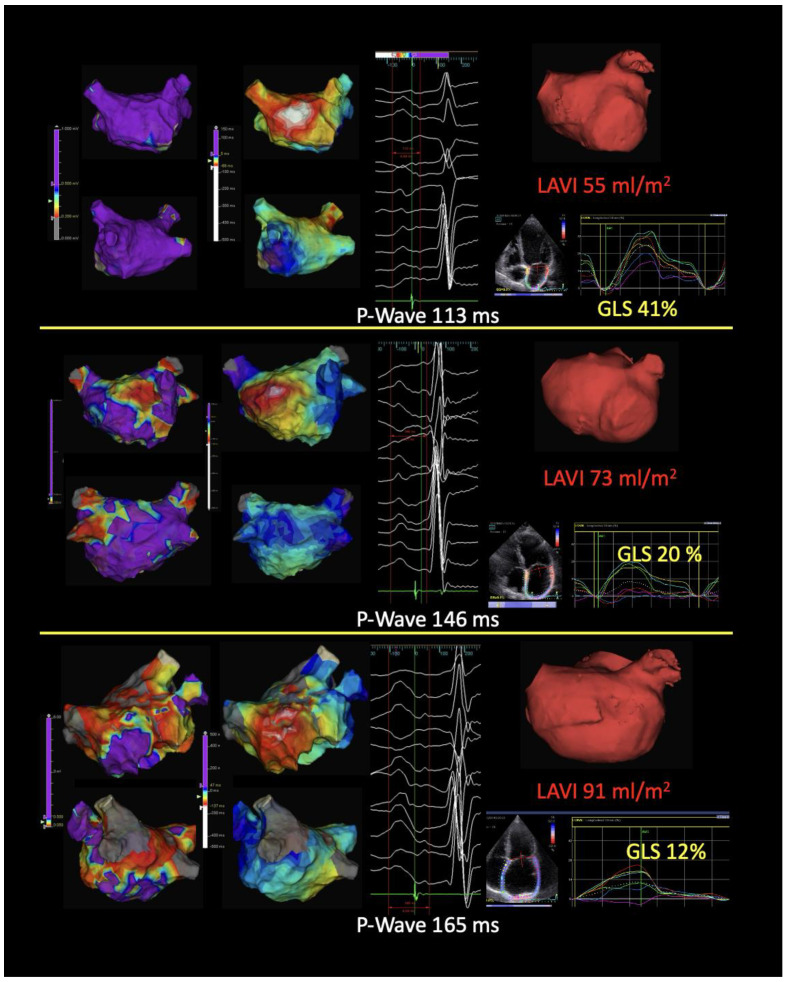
A multimodal approach may contribute to detection of left atrial low voltage area. In these examples from the left to the right: voltage map during sinus rhythm (cutoff < 0.5 mV) and activation map performed with electroanatomical mapping (Ensite SJM), P wave duration, LA dimension detected by CT scan, and deformation detected by echo strain. On top, a case of normal voltage with normal conduction; the P wave is not prolonged, and deformation is normal in this case. In the middle, a case of prolonged P wave, LA enlargement at the CT scan, and impaired strain with moderate low voltage. On the bottom, severely dilated LA, P wave prolongation, and a severely impaired LA strain with a severe degree of low voltage area.
